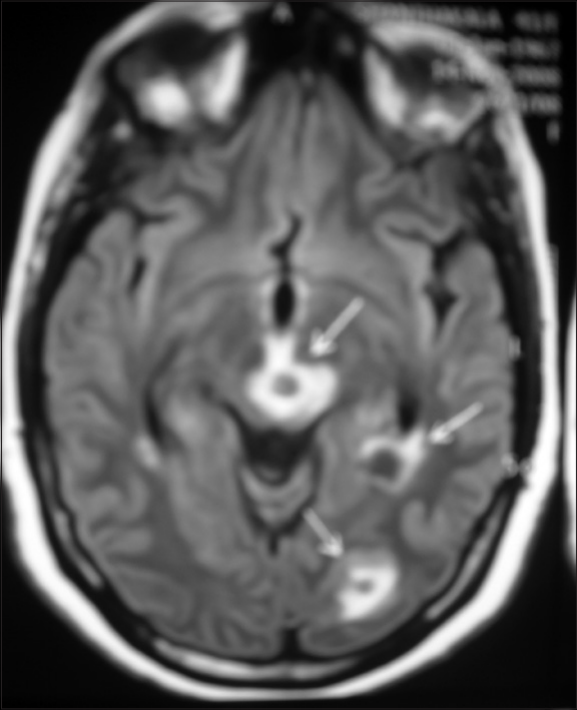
T2 weighted image of MRI brain showing multiple metastatic lesions
